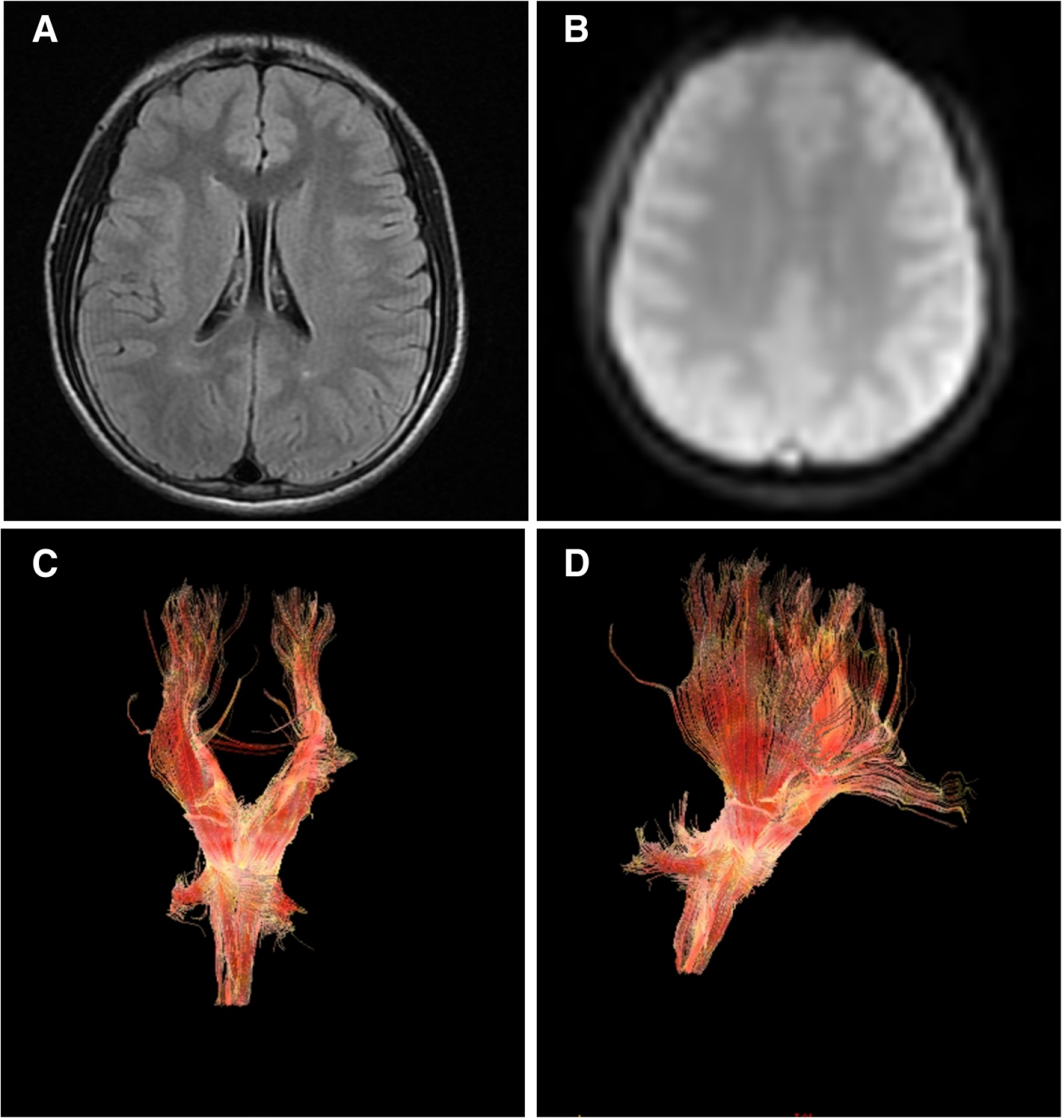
MRI of the head showed normal structures. a Axial FLAIR image at the level of the lateral ventricles. b Diffusion Tensor Imaging (DTI) image. c, d DTI color maps
